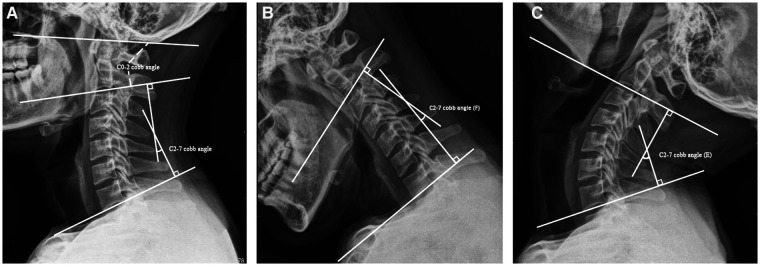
The definition of radiological assessment. (A) The definition of C0–2 and C2–7 Cobb angles. (B,C) The cervical ROM was defined as the difference between the C2–7 Cobb angle of flexion–extension lateral radiographs. ROM, range of motion.
